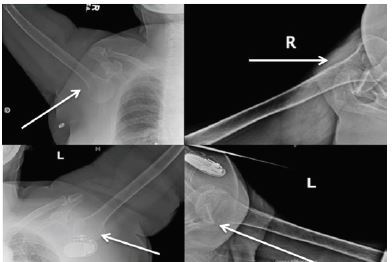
Radiographs demonstrating bilateral inferior shoulder dislocations without fracture. White arrows pointing to humeral head of bilateral shoulders, which is directly inferior to the glenoid.
